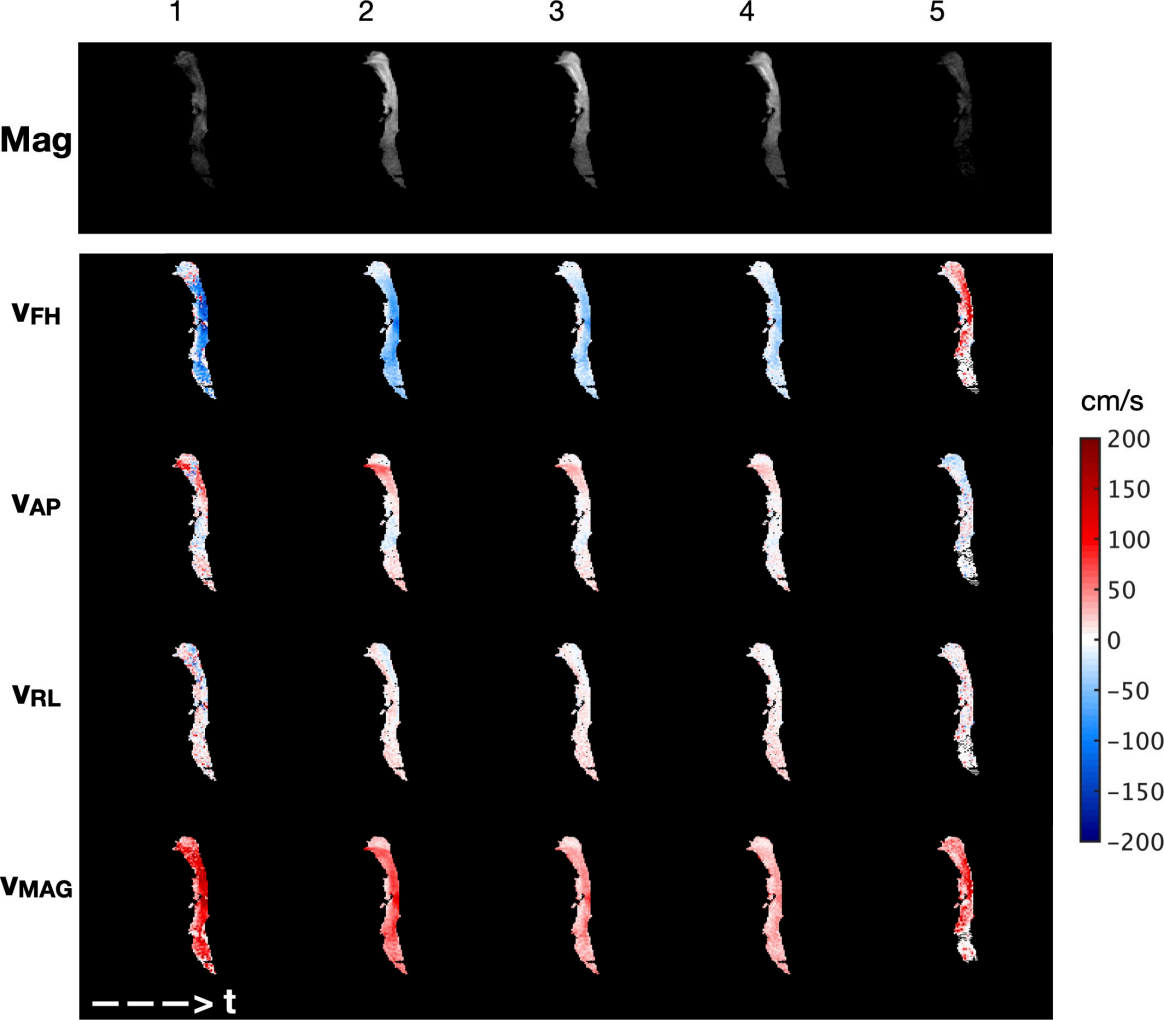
Example ^129^Xe gas PC MRI dataset acquired from subject 1. The first 5 dynamic images from a 10-image time series are shown left-right, where each dynamic scan corresponds to a 3.1 s interval. Top row: MRI magnitude images showing the ^129^Xe gas density in the airway. Lower rows: Foot-head (*v*_*FH*_), anterior-posterior (*v*_*AP*_) and right-left (*v*_*RL*_) components of velocity derived from the dynamic PC MRI time series, and the corresponding velocity magnitude (*v*_*MAG*_, vector sum of the three directional components). Colour key: For *v*_*FH*_, red = flow from foot to head, blue = flow from head to foot; for *v*_*AP*_ red = flow from anterior to posterior, blue = flow from posterior to anterior; and for *v*_*RL*_ red = flow from right to left, blue = flow from left to right. *v*_*MAG*_ is non-directional.
